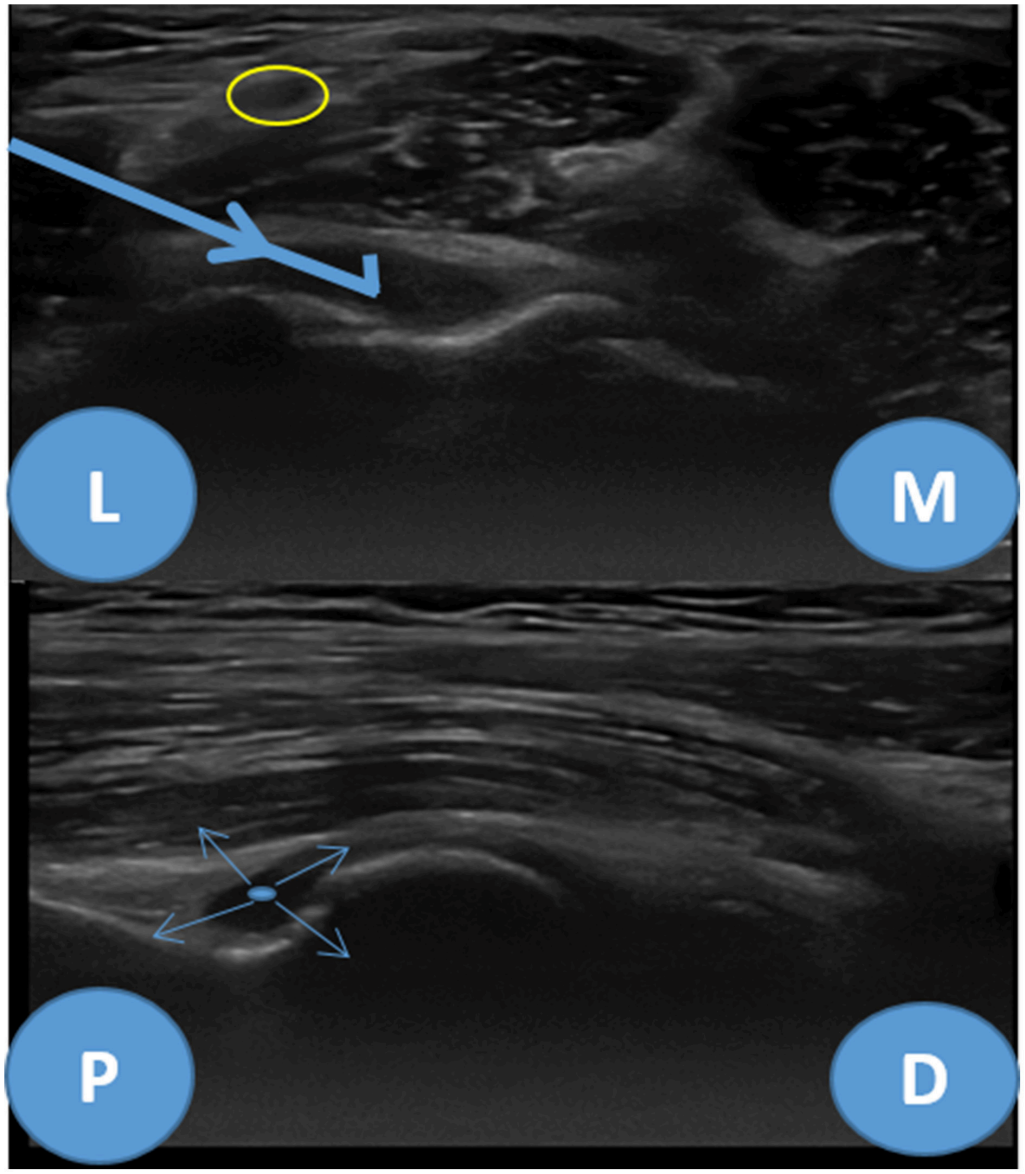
Anterolateral approach for elbow biopsy, ultrasound images. Upper image—transverse view, L, lateral; M, medial. Yellow circle—radial nerve, Bold blue arrow—biopsy needle trajectory. Lower image—longitudinal view, P, proximal; D, distal. Thin blue arrows—needle throw directions.
